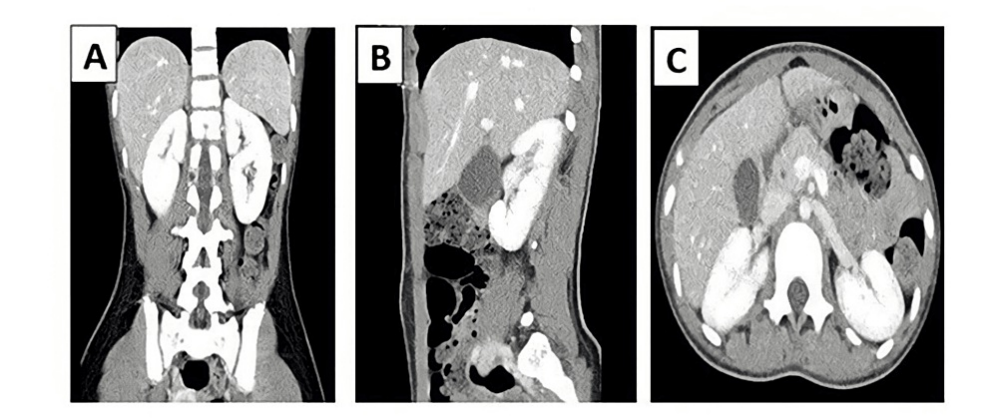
Coronal (A), sagittal (B), and axial (C) views of repeat abdominal computed tomography scan with intravenous contrast. Coronal, sagittal, and axial views of repeat abdominal CT scan show complete resolution of renal lesions following successful treatment of MIS-C.
